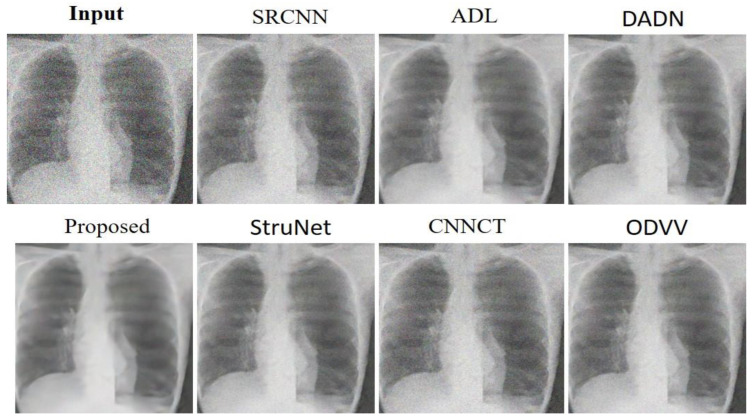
Results were gathered from the noise-removal models applied to the Chest X-ray dataset.
